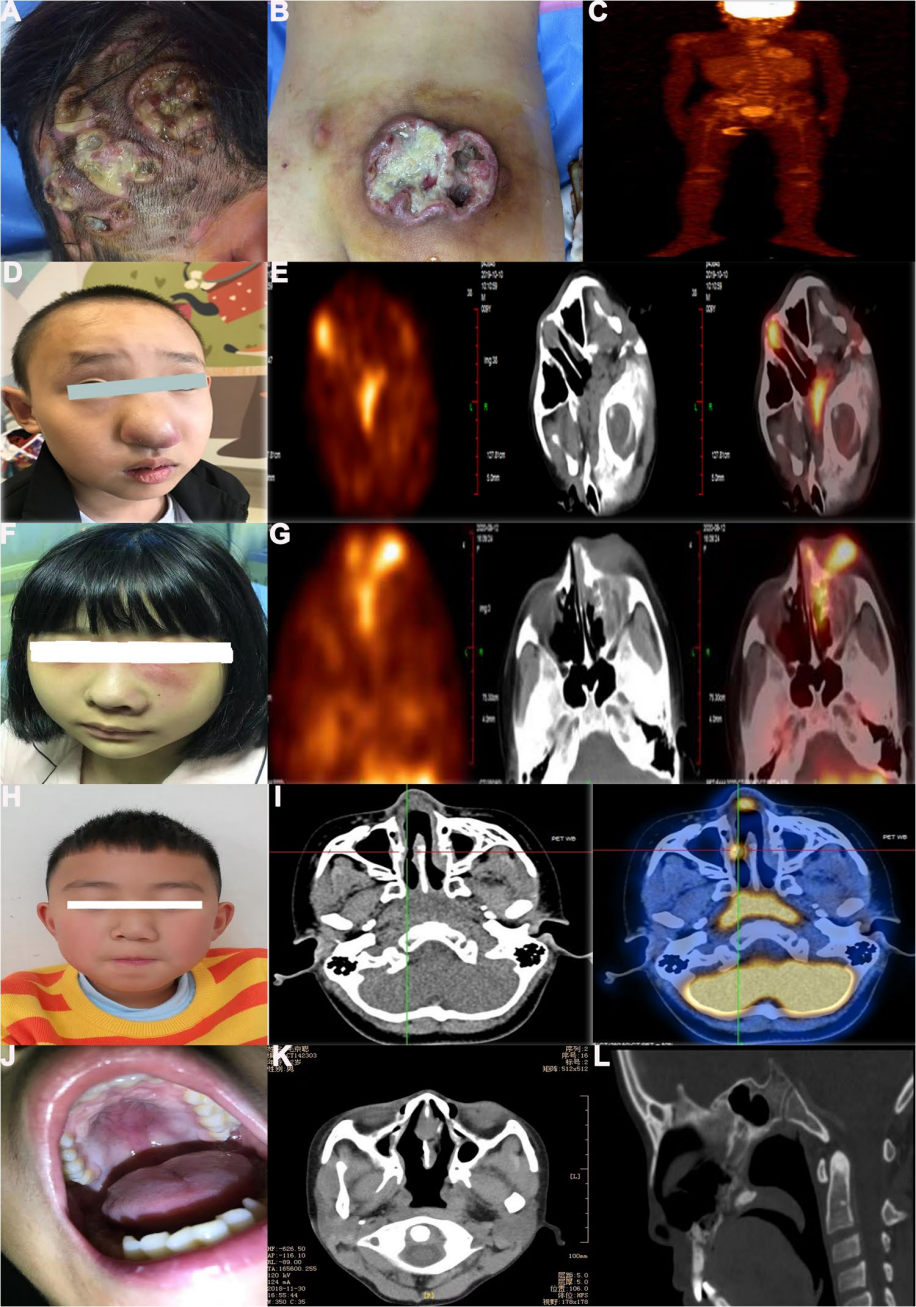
Case 1: Skin ulcers with yellow and white secretions covered on the head (A) and right lumbosacral region (B). PET/CT revealed that tumor involved in multiple skin and subcutaneous tissues, nose, bone, bilateral cervical and inguinal lymph nodes (C). Case 2: Alae nasi of the patient was red and swollen (D). The PET imaging demonstrated that tumor involved in nose, anterior nasal cavit, palatine tonsil, adenoid (E). Case 3: The patient had left maxillofacial redness and swelling, left alar nasi swelling (F). Serum EBV-DNA load was 1.91 × 10^3^copies/ml. The PET imaging showed that tumor involved in the left maxillofacial region, nasal root, inner canthus of the left eye and left middle and lower nasal meatus (G). Case 4: The patient had the left nasal alar and left nasal mass (H). The PET imaging showed the tumor involved in the posterior nasal septum and the right nasal cavity (I). Case 5: Mucosa of the midline upper jaw was red and swollen (J). Nasopharynx enhanced CT imaging showed that soft tissue thickening of nasal septum and lateral nasal wall, partial discontinuity of nasal septum bone (K), and irregular bone destruction in hard palate and upper alveolar (L)
